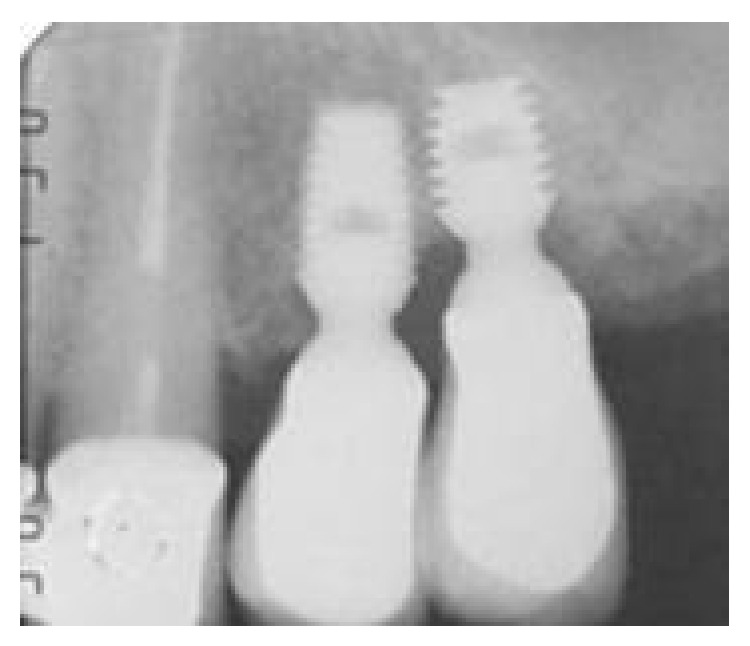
One-year postoperative radiographs depicting radiopacity at the location of the defect.
